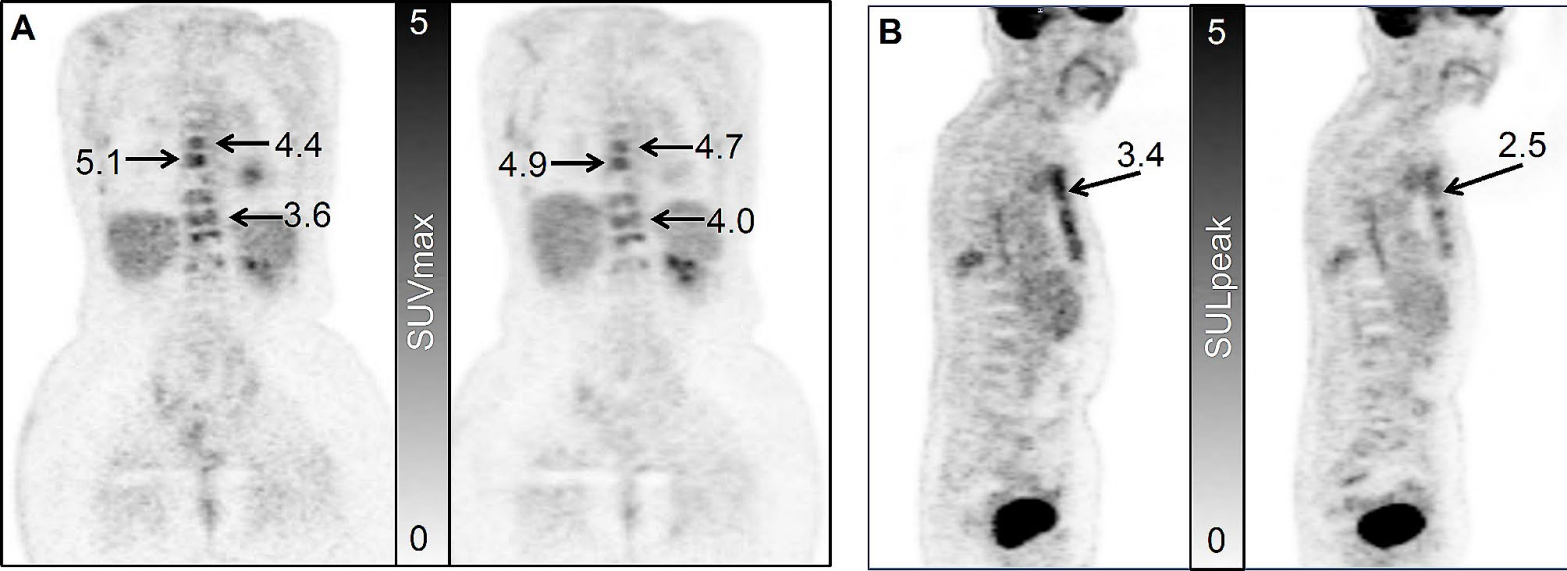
(A) Cohort-1 study. Coronal ^18^F-FDG-PET images from the same patient imaged 7 days apart with SUVmax values indicated. (B) Cohort-2 study. Sagittal ^18^F-FDG-PET images of a 90-year old female with bone-dominant MBC. Left: Pre-therapy baseline scan showing the SULpeak of the index lesion. Right: Post therapy 4mo scan, that shows a decrease of 25%, which met our LRC threshold of -18%, but not the PERCIST threshold of -30%. The SUVmax of the index lesion decreased by 22%. The response was considered stable disease by the criteria developed in this report
